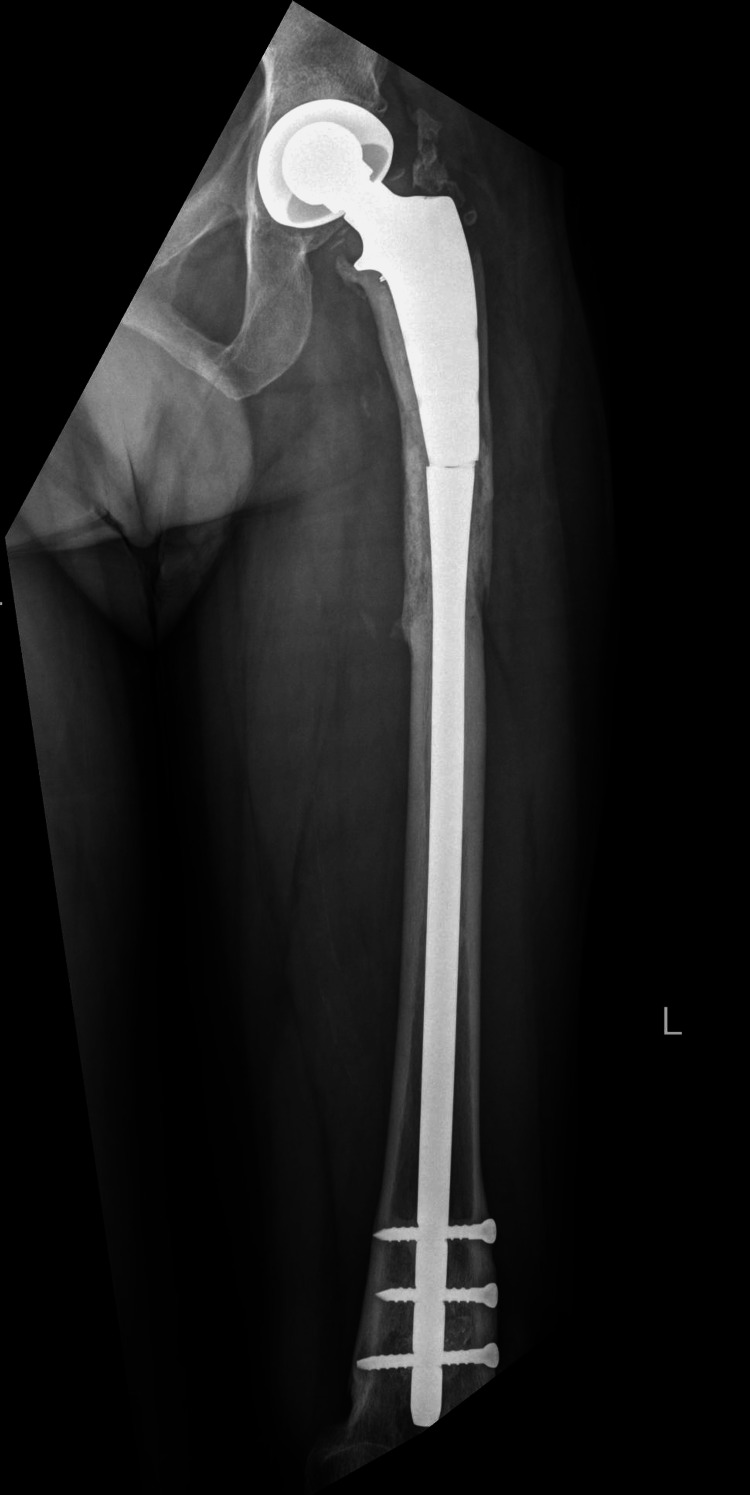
Fourteen years after index surgery radiograph showing implant failure
